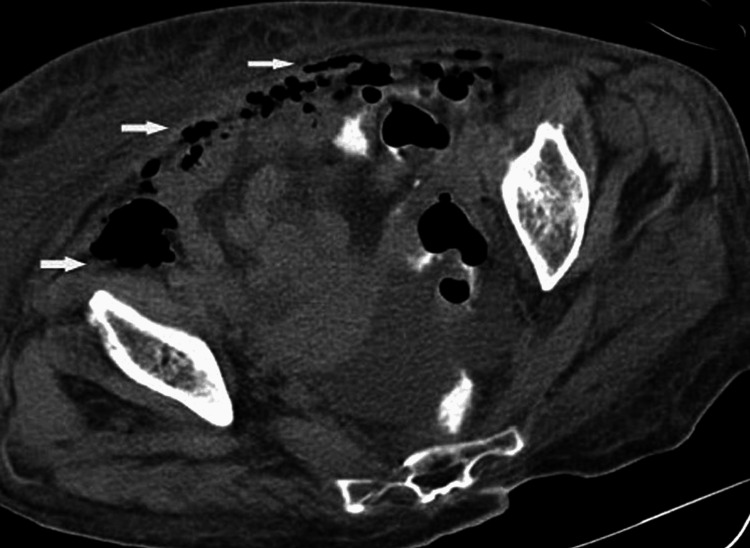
Scan of a 70-year-old female who developed diabetic ketoacidosis An axial CT image shows free air (arrows) in the abdomen and pelvis. Extensive anasarca edema is present.
